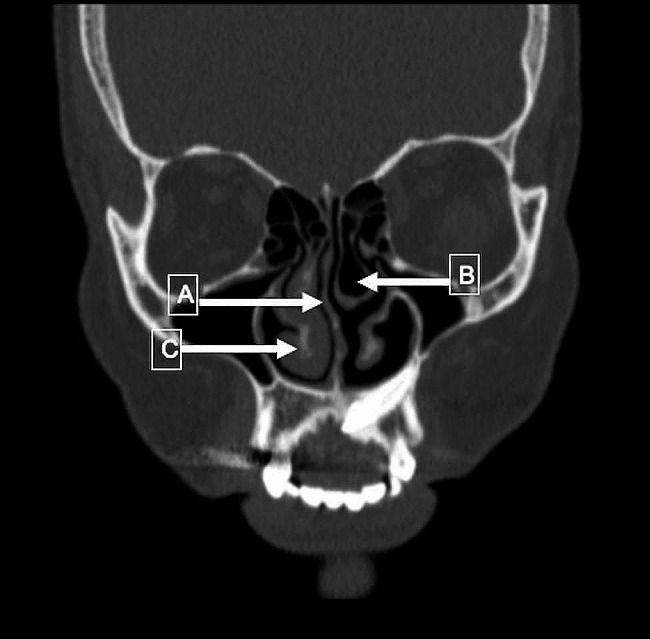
Coronal CT image of the patient who underwent external dacryocystorhinostomy for acquired nasolacrimal duct obstruction. (A) nasal septal deviation, (B) concha bullosa, (C) inferior turbinate hypertrophy
